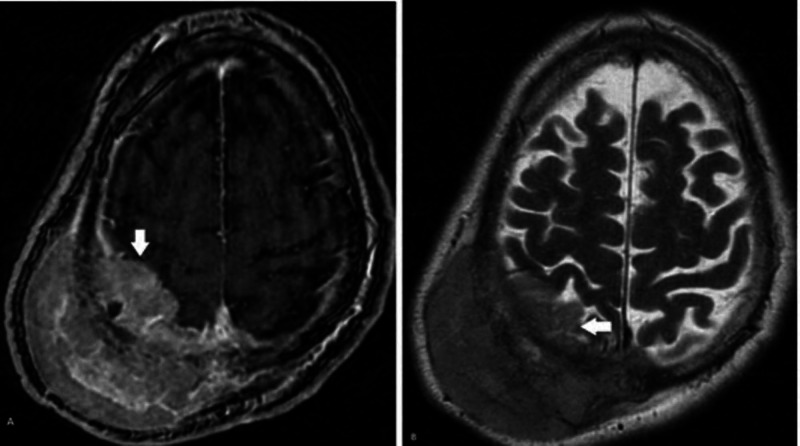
MRI brain with and without contrast demonstrating a somewhat heterogeneously enhancing mass with dural involvement and mild associated parenchymal edema A. axial post-contrast T1-weighted image, B. axial T2-weighted image MRI: magnetic resonance imaging
